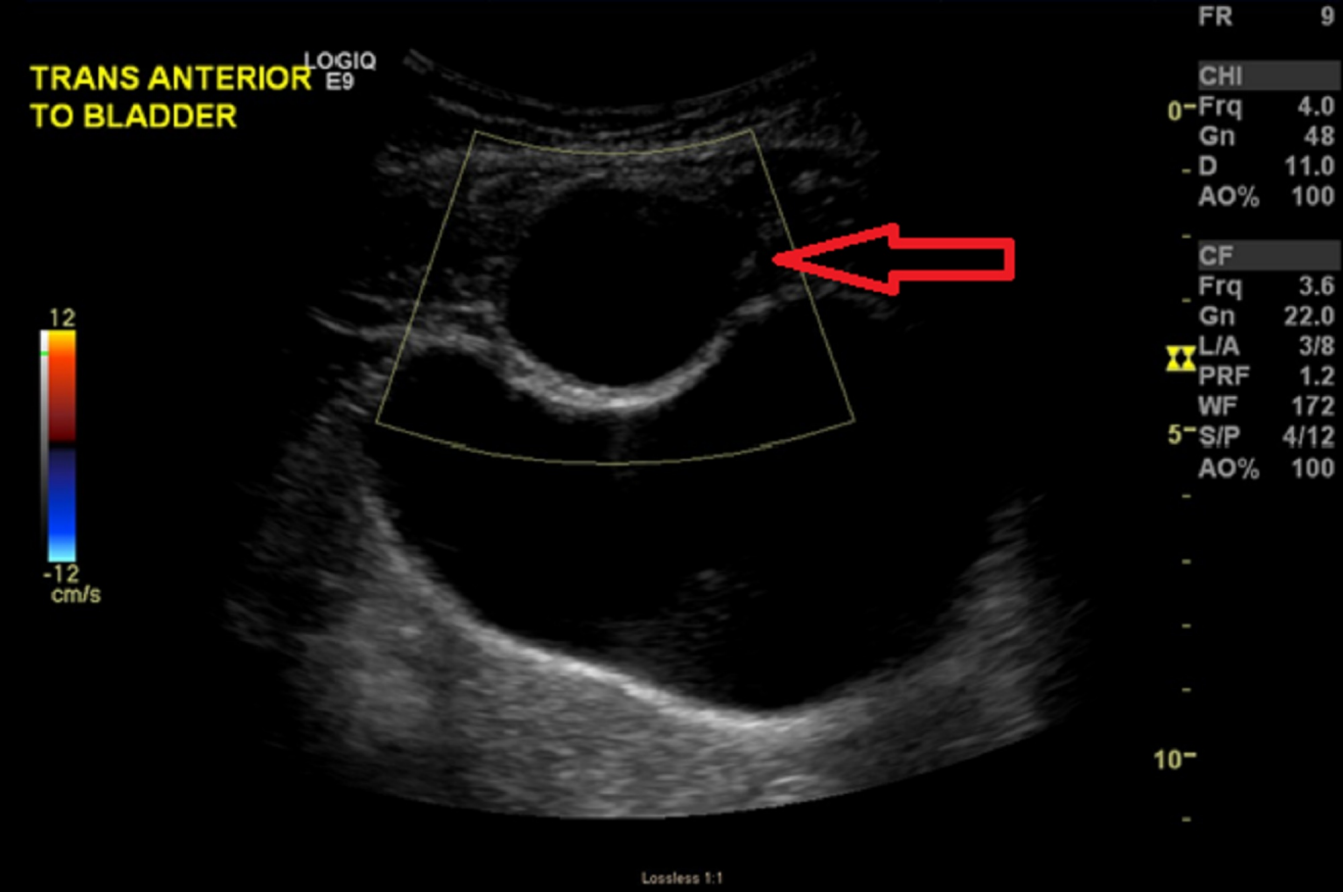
Sonography and Doppler examination of the abdomen revealing a 5 cm x 3 cm heterogeneous hypoechoic mass (arrow)
